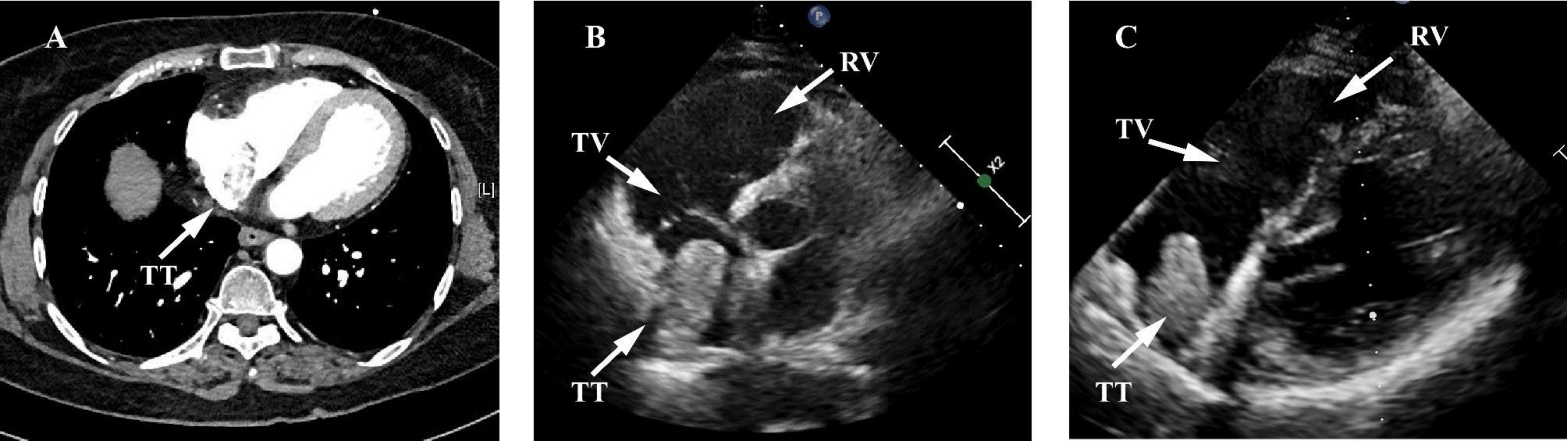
The preoperative imaging findings of renal cell carcinoma patients with right atrial tumor thrombus. (A) Contrast-enhanced computed tomography demonstrating the tumor thrombus (white arrow) in the right atrium. (B, C) The ultrasonography showing the tumor thrombus floating in the right atrium. RV, right ventricle; TT, tumor thrombus; TV, tricuspid valve
